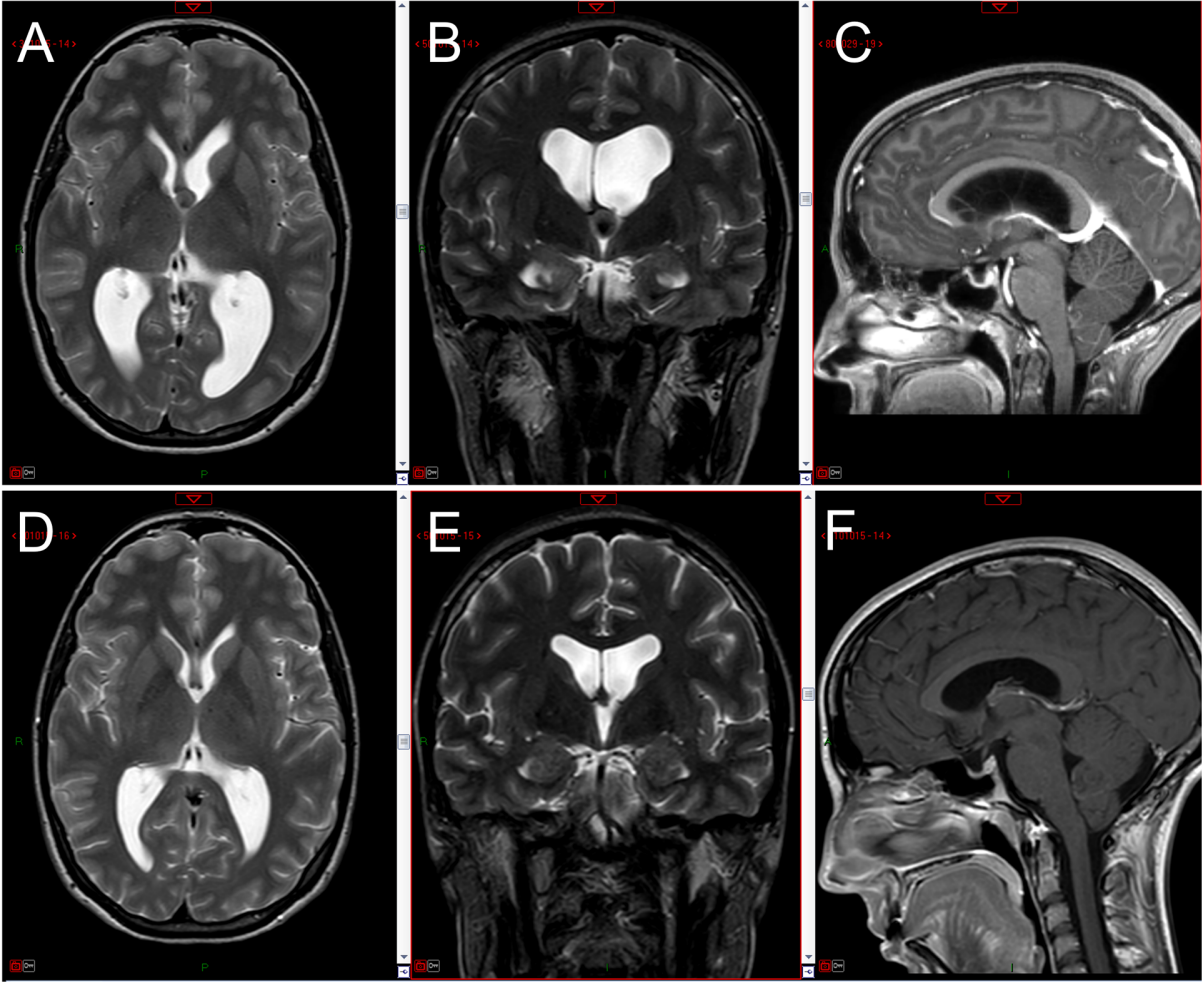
Preoperative MRI images (axial and coronal T2-weighted, sagittal enhanced T1 weighted) showing the third ventricle colloid cyst with bilateral (left > right) ventriculomegaly (A–C); three-month postoperative MRI images showing complete removal of the cyst with marked reduction in ventricular size (D–F).
